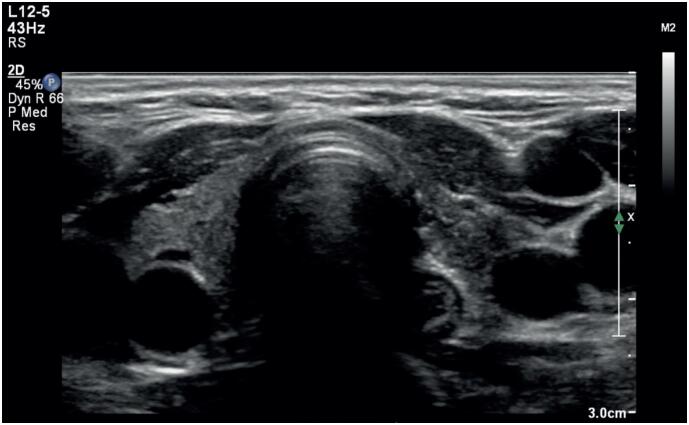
Ultrasonography of the thyroid gland reveals a marked reduction in size, indicating an overall atrophic appearance (total thyroid volume, 4.2cm^3^; normal range, 6-15cm^3^) in a 37-year-old woman with atrophic hypothyroidism associated with elevated thyrotropin receptor antibodies
